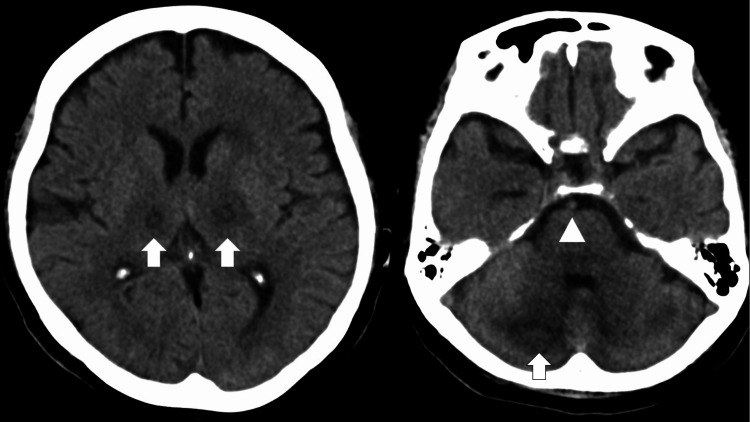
Brain CT on the second day. Low-dense areas in the bilateral thalamus and the cerebellum (white arrow) and a hyperdense right middle cerebral artery sign (white arrowhead) were seen.
